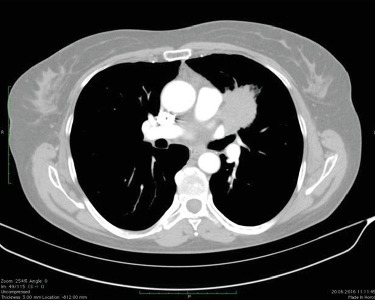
The CT scan showed new target lesion situated paravertebrally in the left lung.
